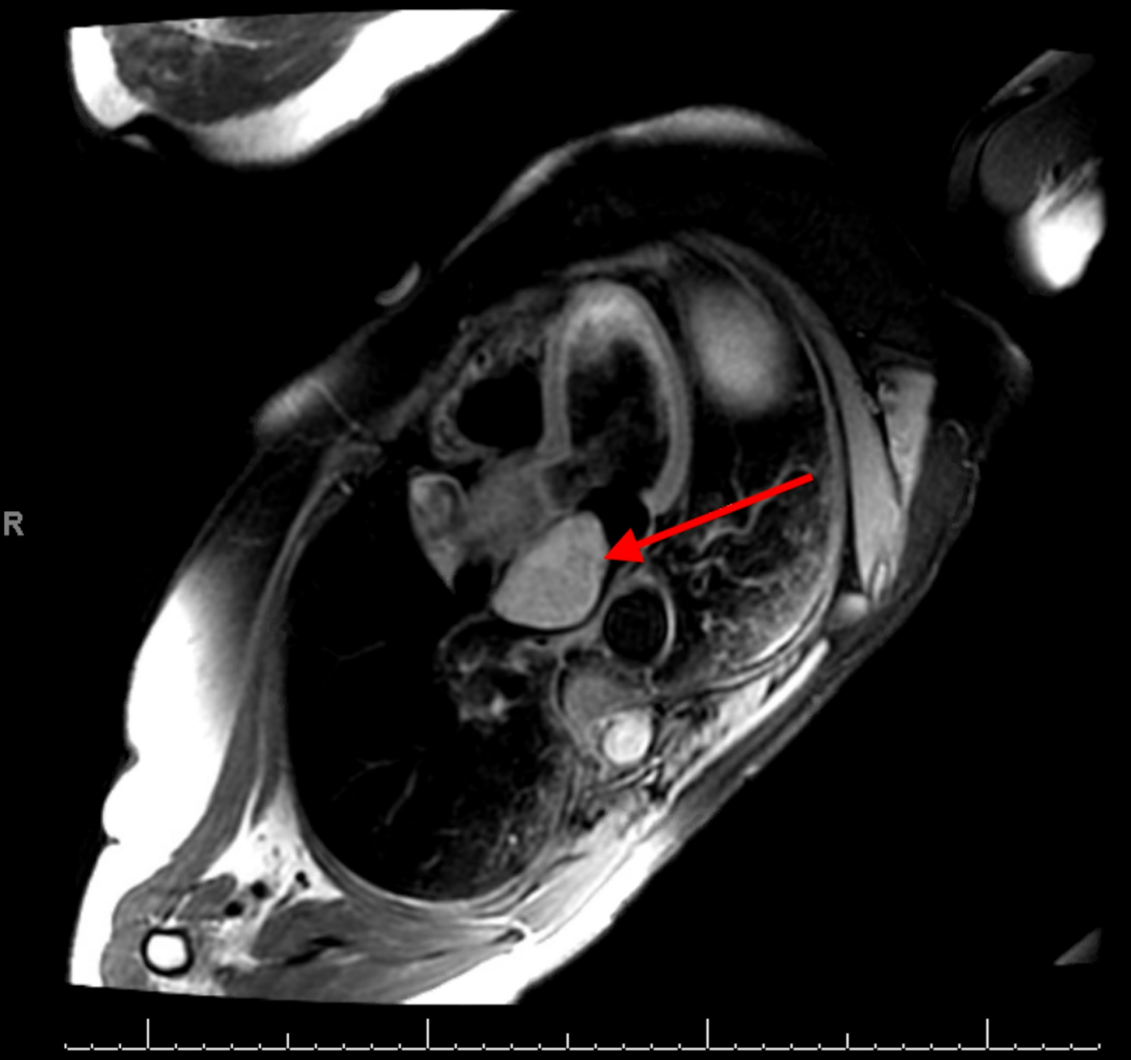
Cardiac magnetic resonance image depicting left atrial mass (red arrow)
